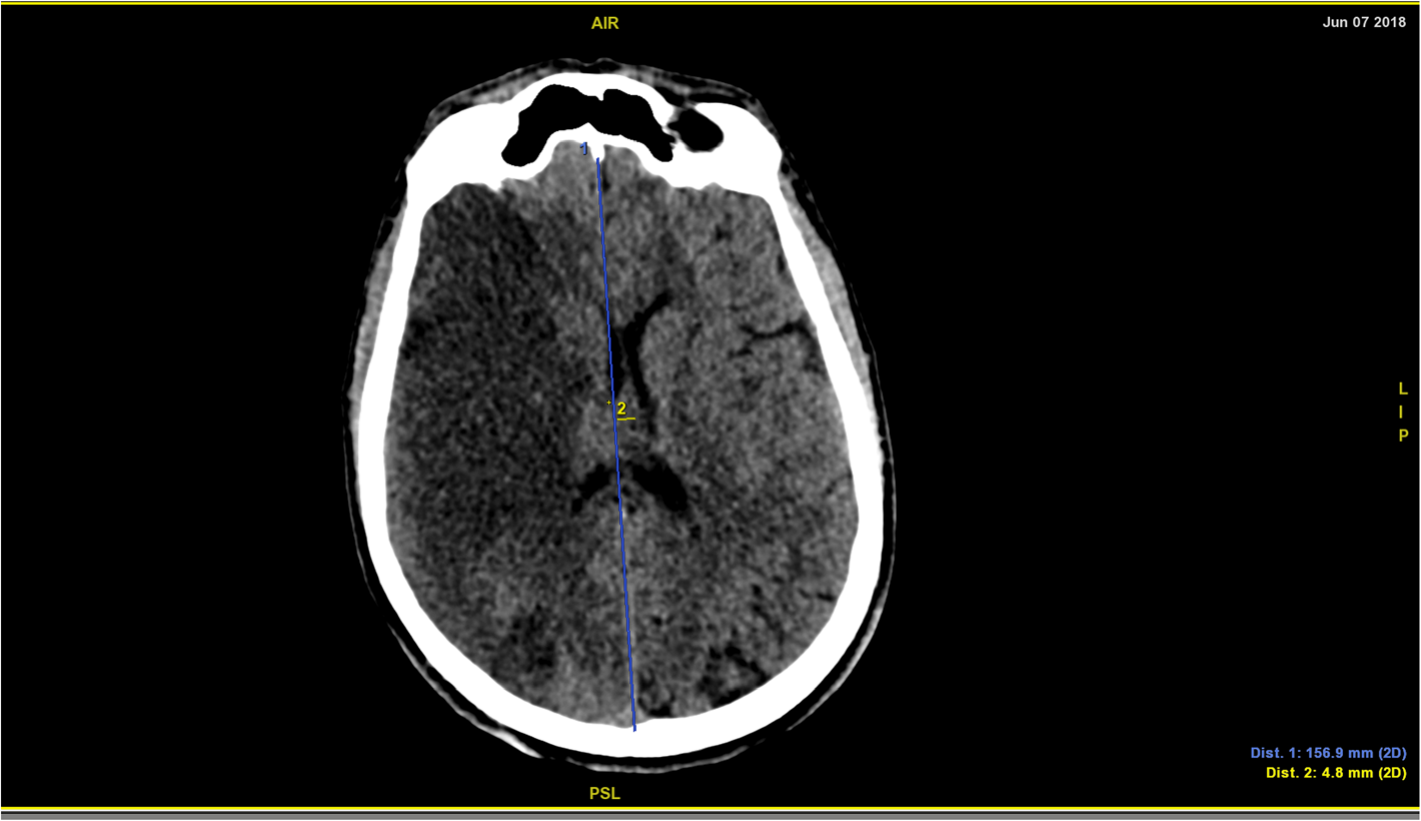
A brain computed tomography scan without contrast revealed a right sylvian ischemic stroke with a mass effect and a midline shift of 4.8 mm
